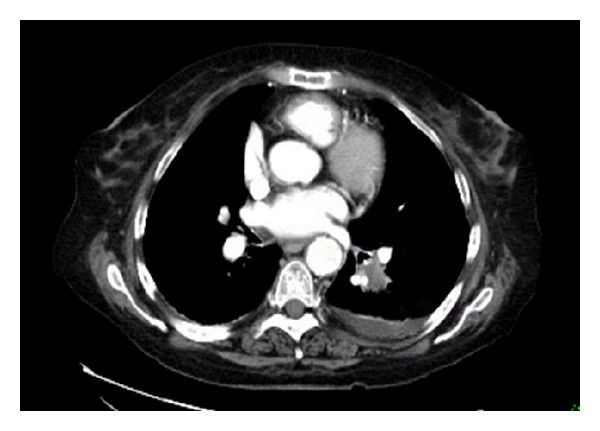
CT scan of the chest showing a spiculated mass in left lower lobe of the lung.
